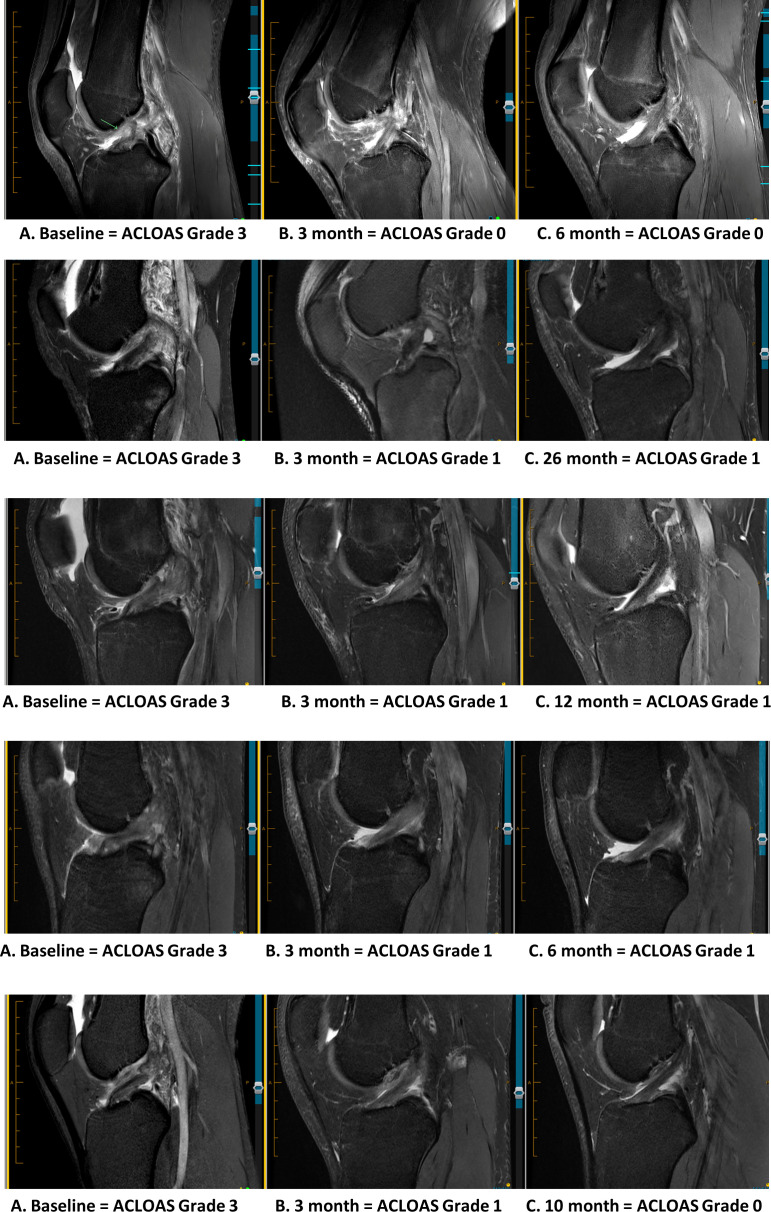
MRI images demonstrating MRI evidence of ACL healing for five participants. ACL, anterior cruciate ligament; ACLOAS, Anterior Cruciate Ligament OsteoArthritis Score.
